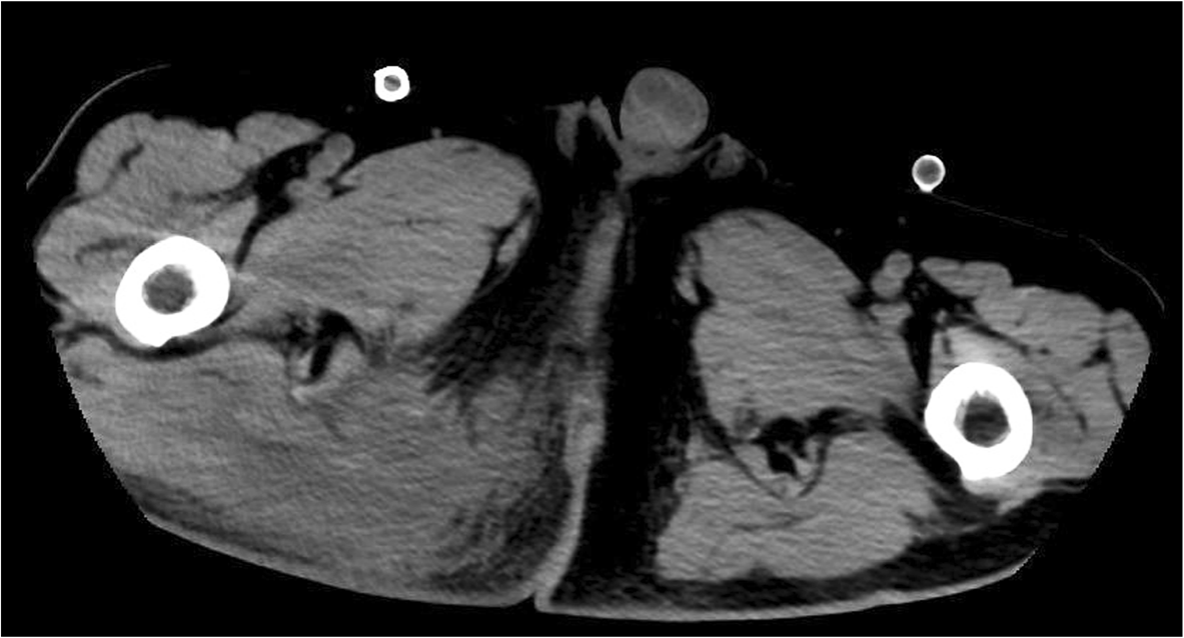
Computed tomography image of the pelvis showing a large low-density area in the right gluteus maximus muscle
